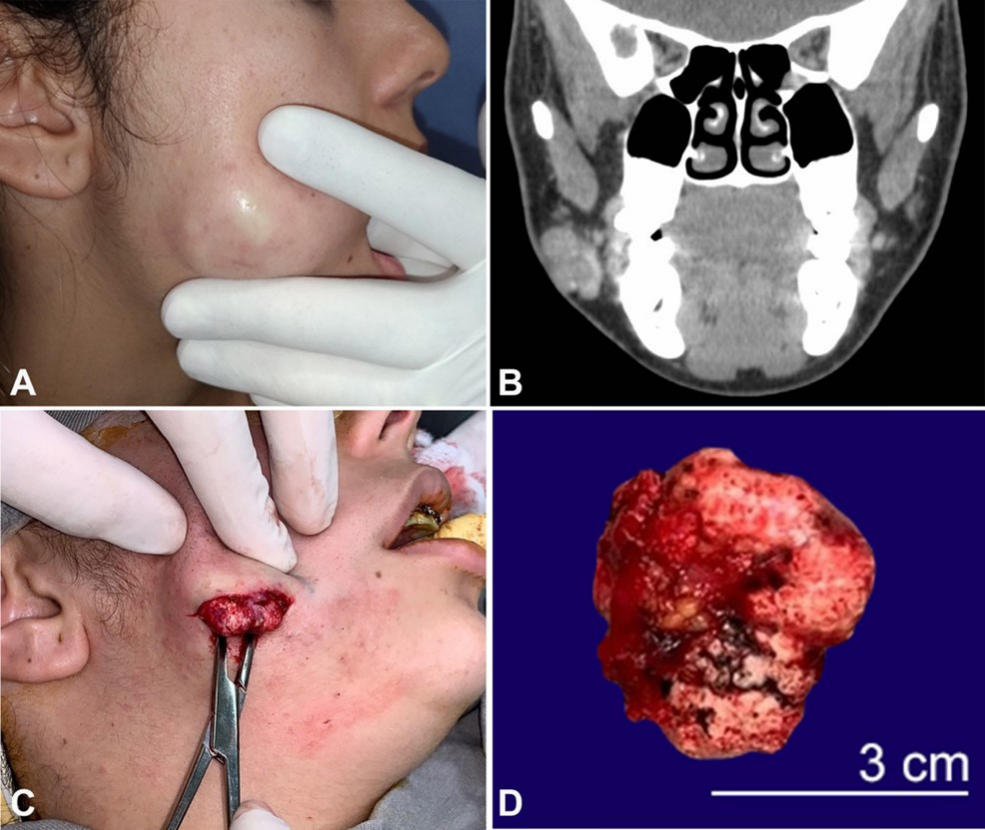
A – Clinical aspect of the lesion. Details for regions of discoloration (ischemia) of the skin when the lesion is delimited and compressed against the buccal mucosa; B – contrasted CT examination showing the presence of a nodule with lobulated contours and tiny calcifications of subcutaneous permeation, reaching a dimension of 2.6 cm; C – dissection was performed using delicate Kelly forceps to separate the lesion capsule from adjacent muscle tissues and skin for total lesion excision in a single fragment; D – Macroscopic aspect of the lesion. A solid, well-defined lesion measuring approximately 24 mm × 14 mm × 12 mm.
